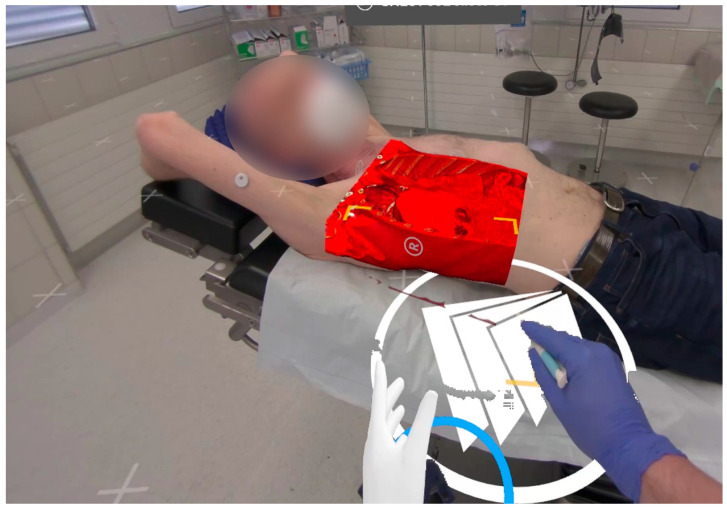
Slicing through overlayed 3D image to examine tumor mass of a solitary fibrous tumor in the right thorax. The right side of the rendered CT image is labeled Ⓡ.
